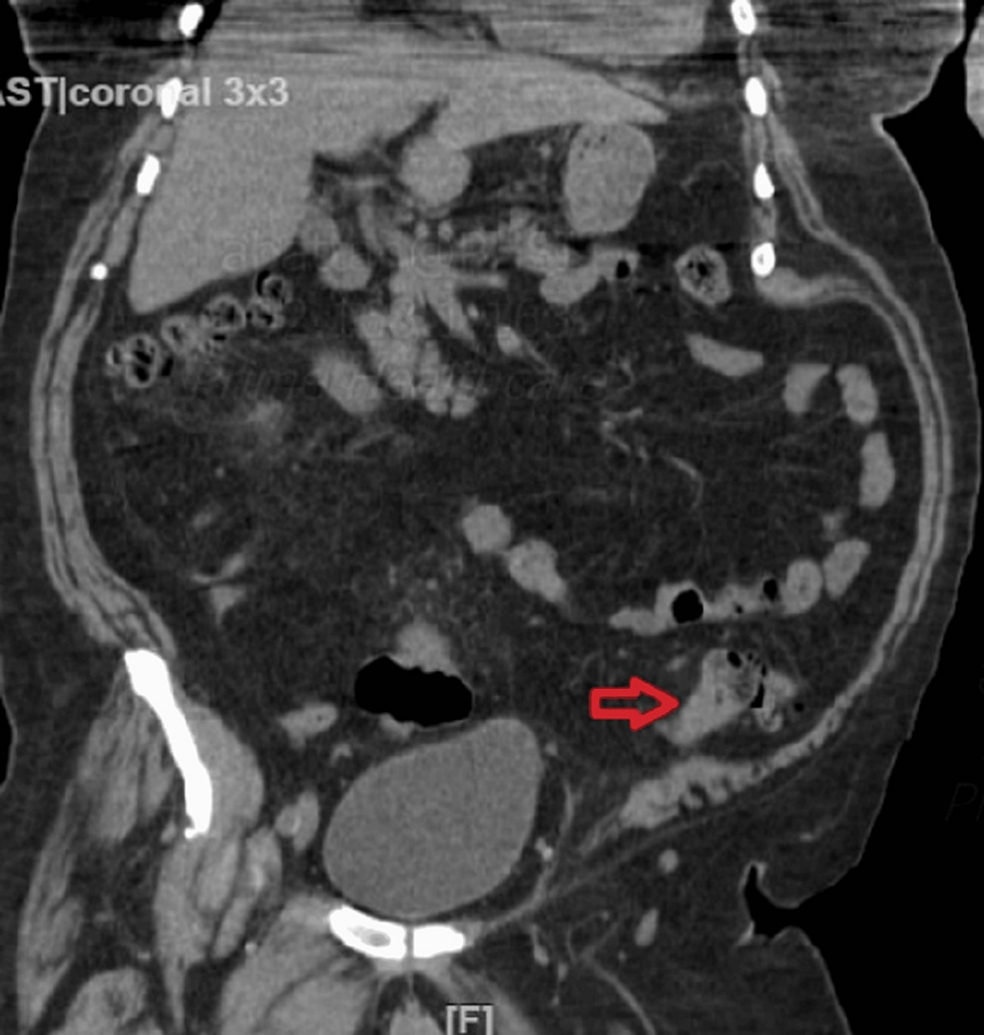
CT abdomen, coronal section, revealed concentric wall thickness of the descending colon (red arrow).
